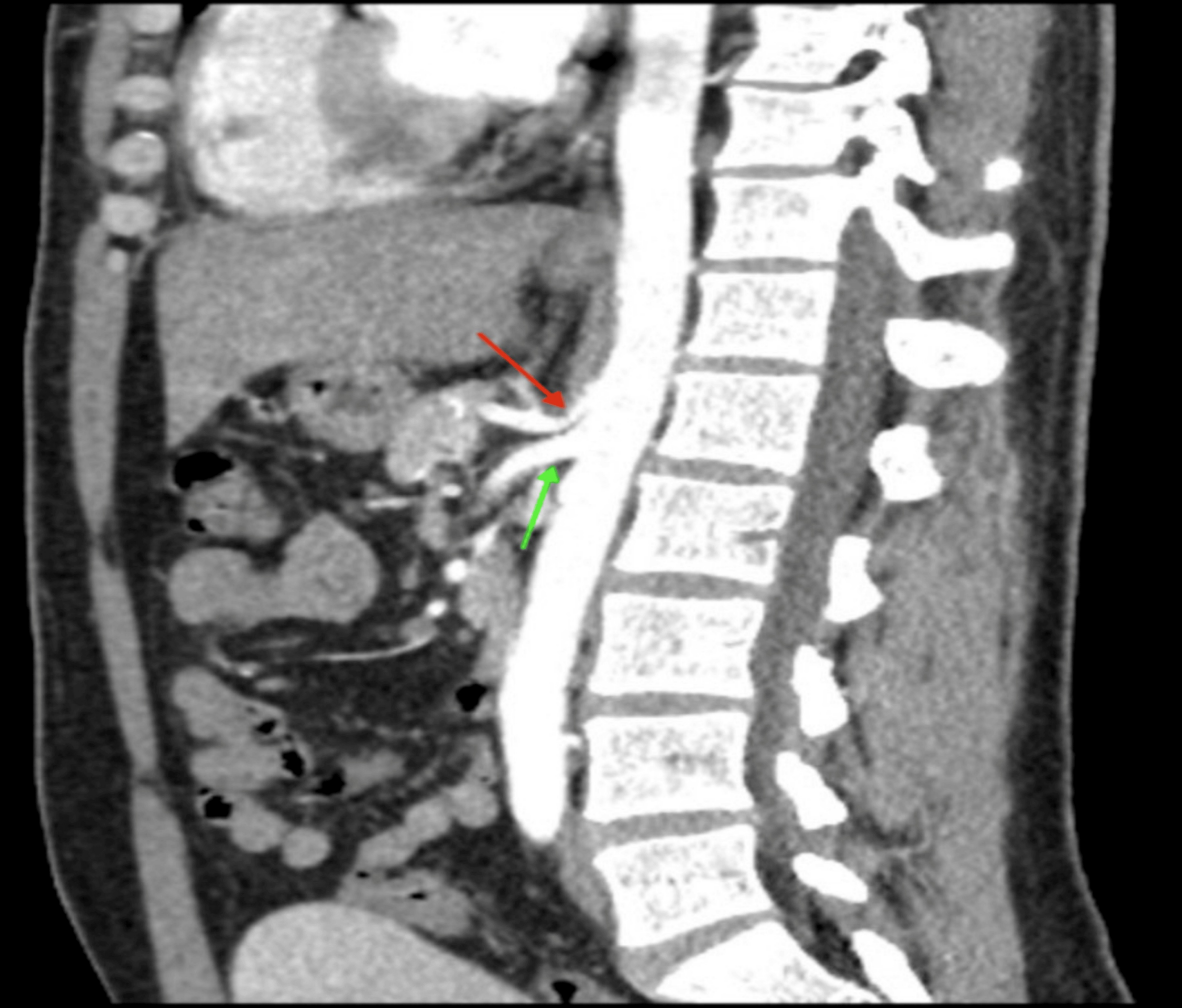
Sagittal Plane View of CTA With Contrast Revealing Stenotic Celiac Artery With Hook Sign (Red Arrow). SMA Appears Patent (Green Arrow) CTA: computed tomography angiography; SMA: superior mesenteric artery
